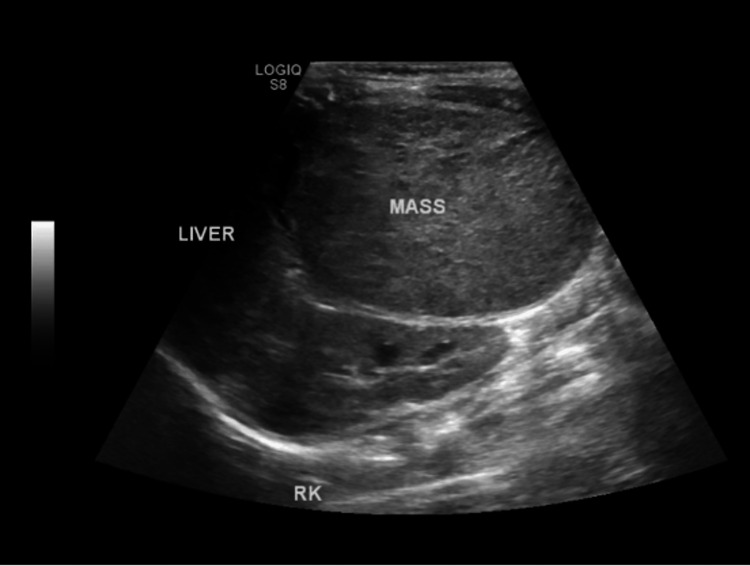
Ultrasound of the abdomen (axial view)- an isoechoic liver mass, measured 9.80 × 9.32 × 6.78 cm.
